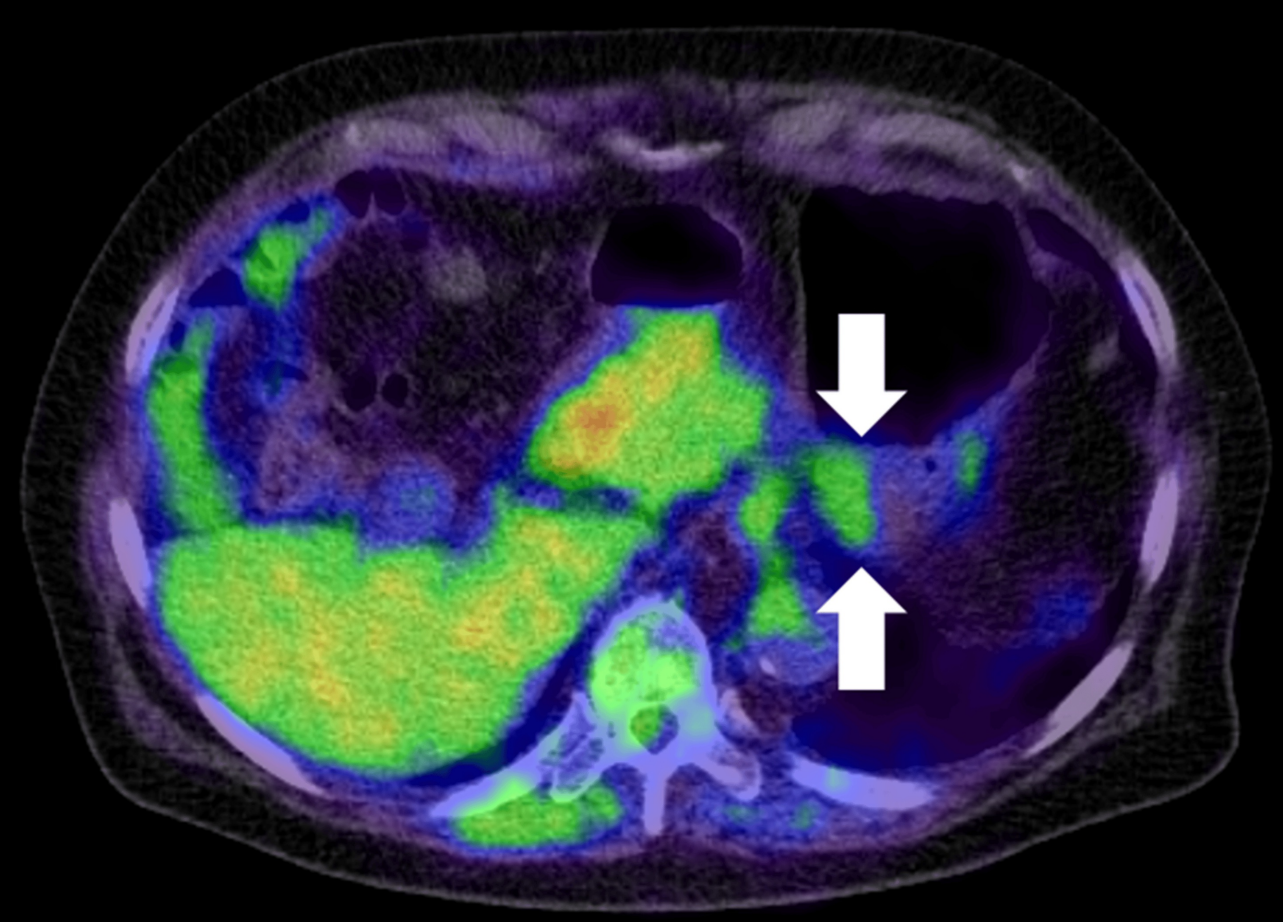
Positron emission tomography (PET) of case 2. The PET showing a maximum standardized uptake value (SUVmax) of 2.92 in the gastric lesion (arrows), which is suggestive of a benign lesion rather than a gastrointestinal stromal tumor.
